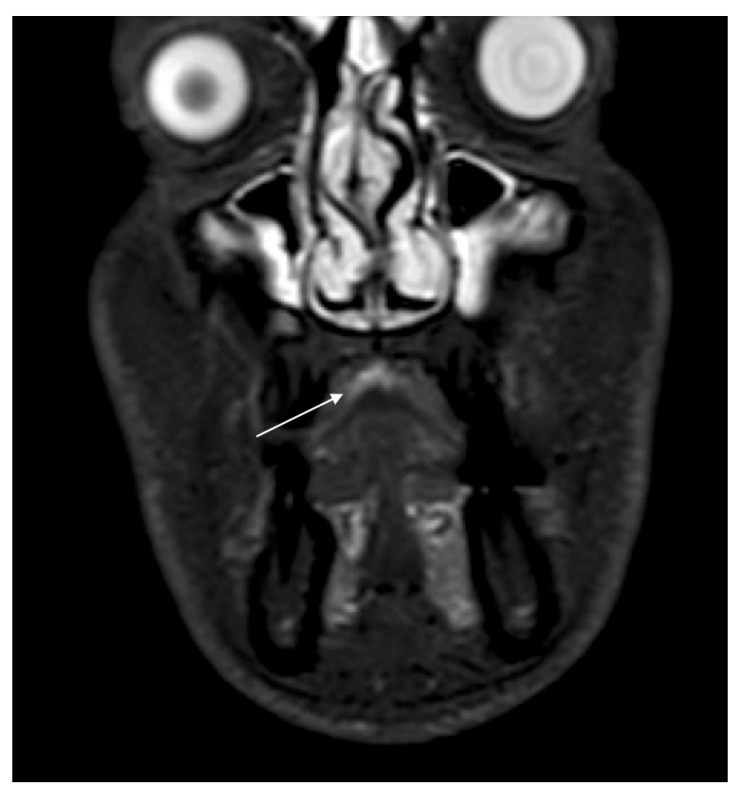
Maxilla–facial MRI in T1WI revealed, in the central area of the hard palate, a thickening of the mucosa of 3–4 mm, with no apparent bone infiltration.
